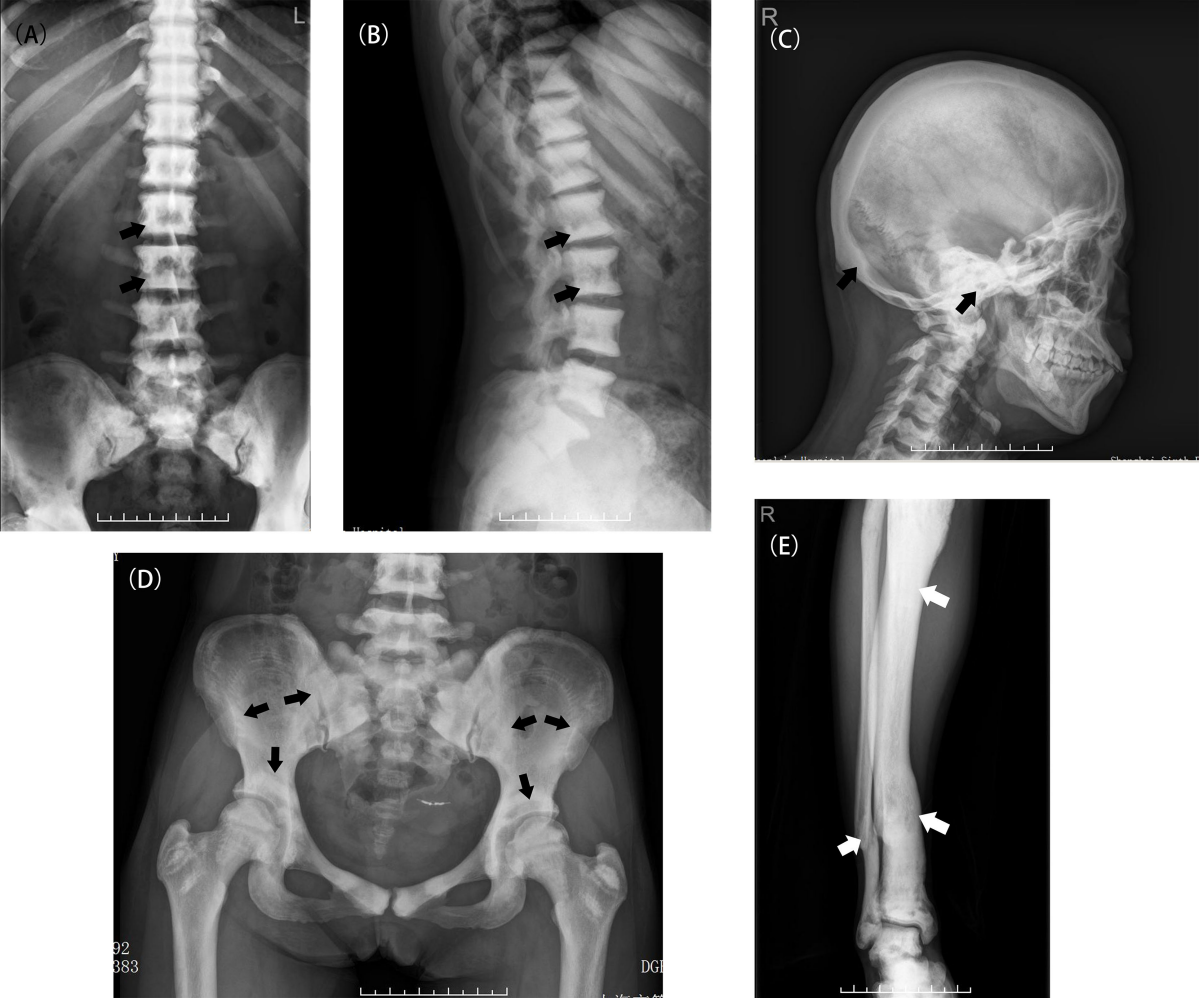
Typical X-ray examinations of ADO II (A, B) A patient (P2) with classic radiograph findings including diffuse osteosclerosis and the appearance of a rugger-jersey spine (black arrow). (C) A patient (P2) with a sclerotic skull, especially in the basis cranii (black arrow). (D) The typical appearance of “bone within bone” in the Patient 5 (black arrow). (E) A deformed tibia due to multiple fractures in the Patient 2(white arrow).
